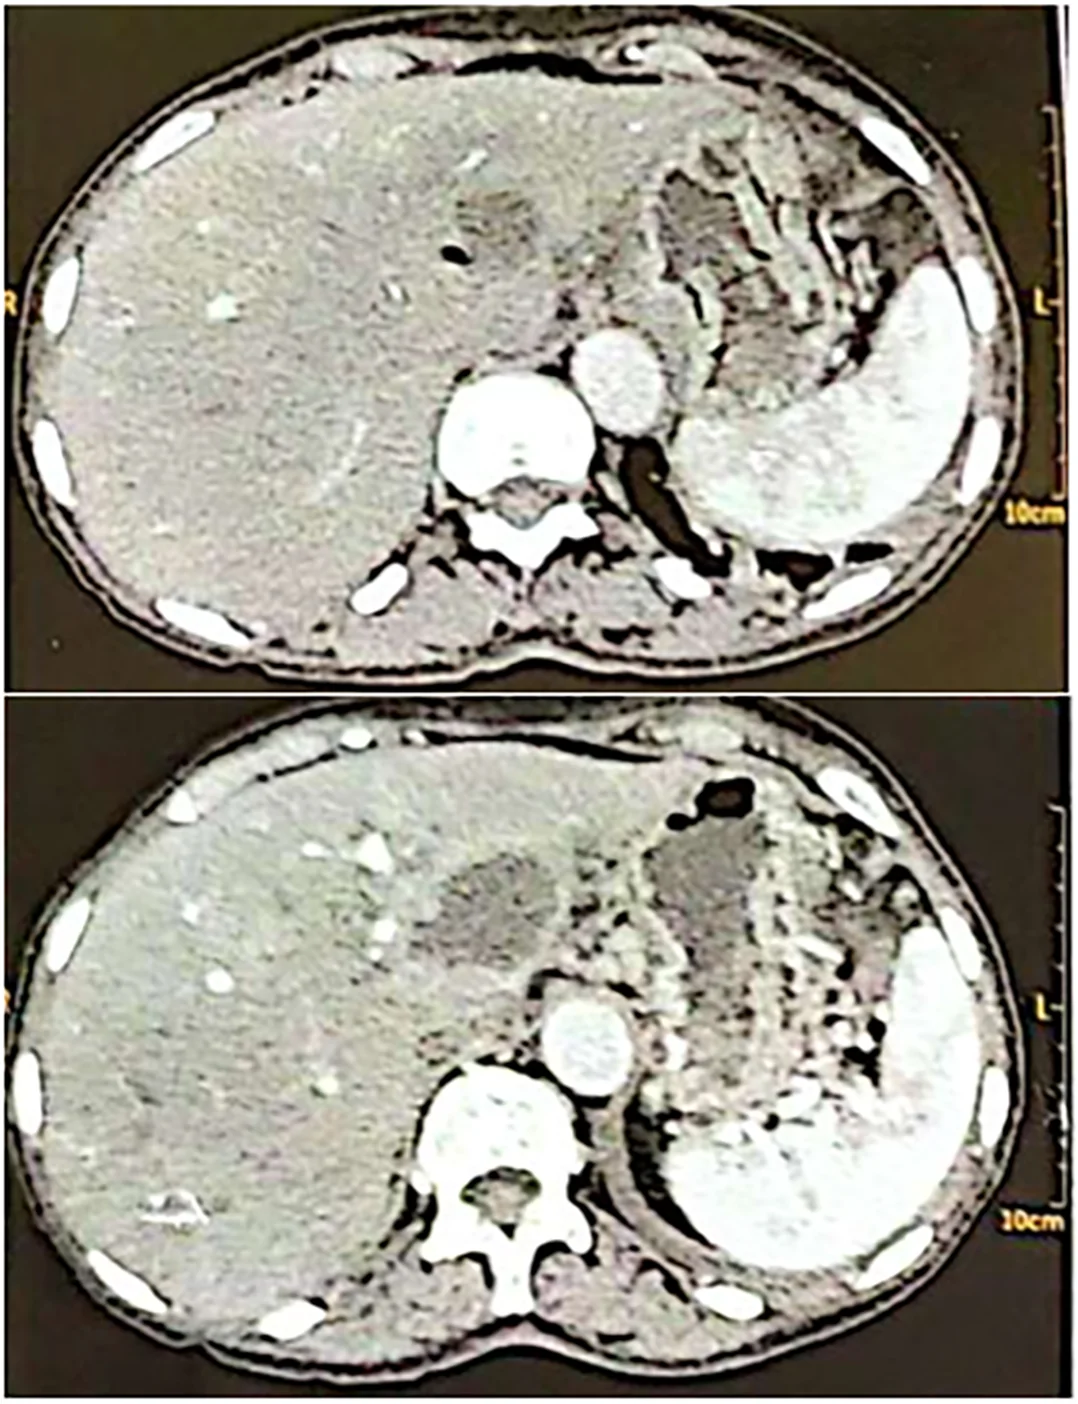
Axial abdominal CT scan with contrast injection.
